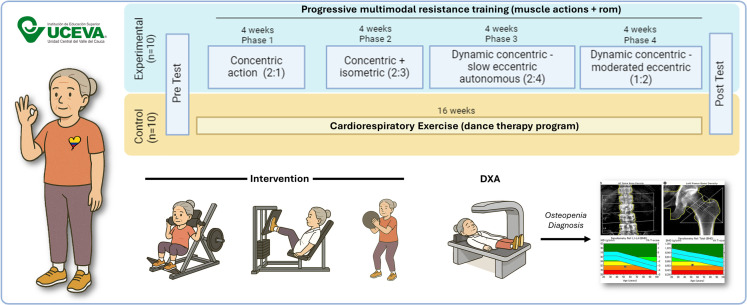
Experimental design of the study. DXA: Dual-energy X-ray Absorptiometry.
